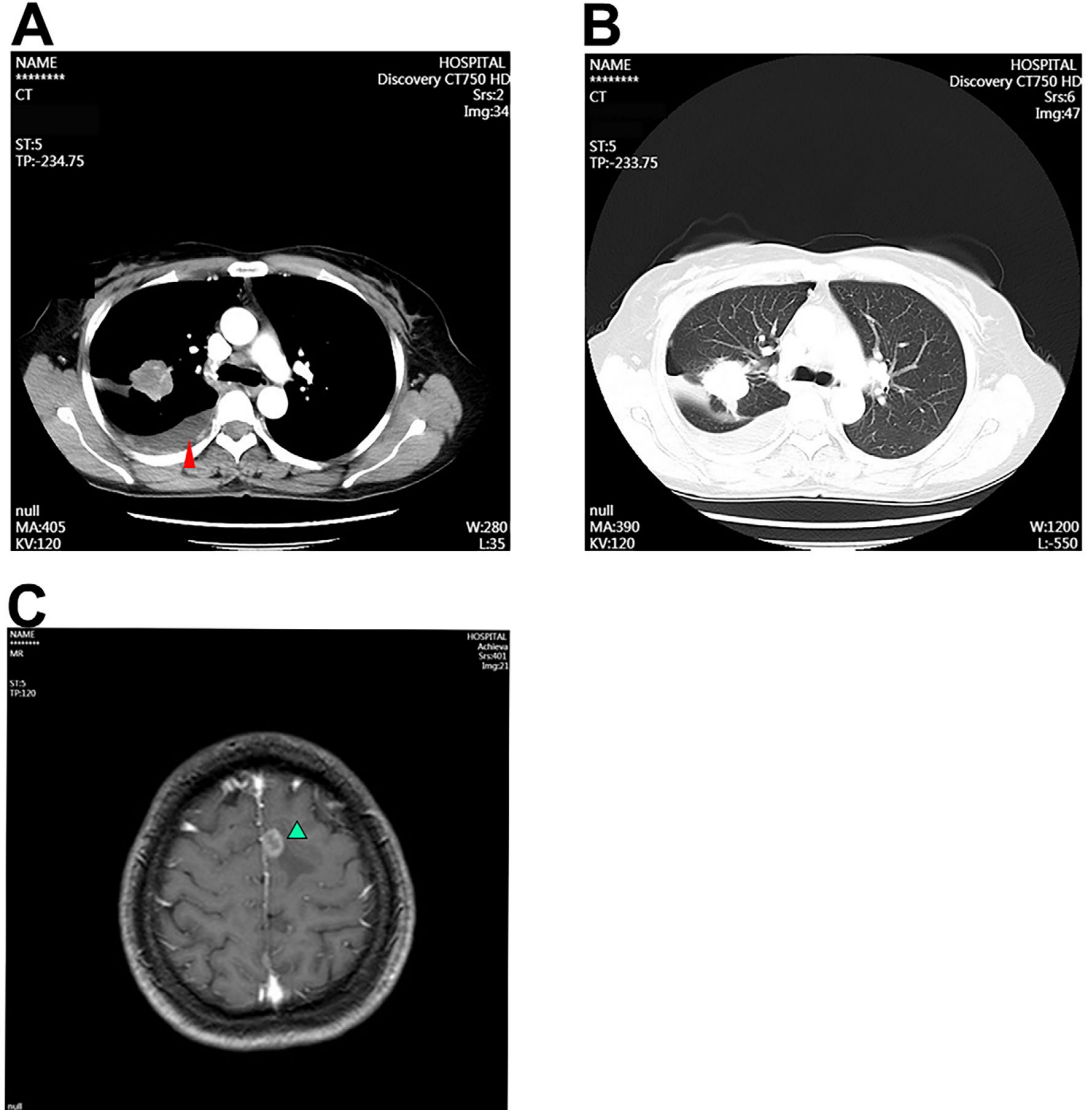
Enhanced CT and head MRI examinations of the patient's chest were performed to assess disease status and progression before treatment. (A, B) The image was captured on July 26, 2022. The high‐density arc‐shaped shadows indicate intrapleural hyperthermic perfusion (red arrowhead). (C) Head MR image of the brain. The green arrows indicate the metastatic focus.
